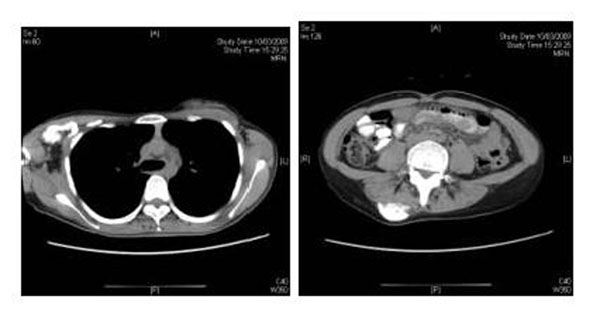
CT images of case 1- extraosseous ossification
